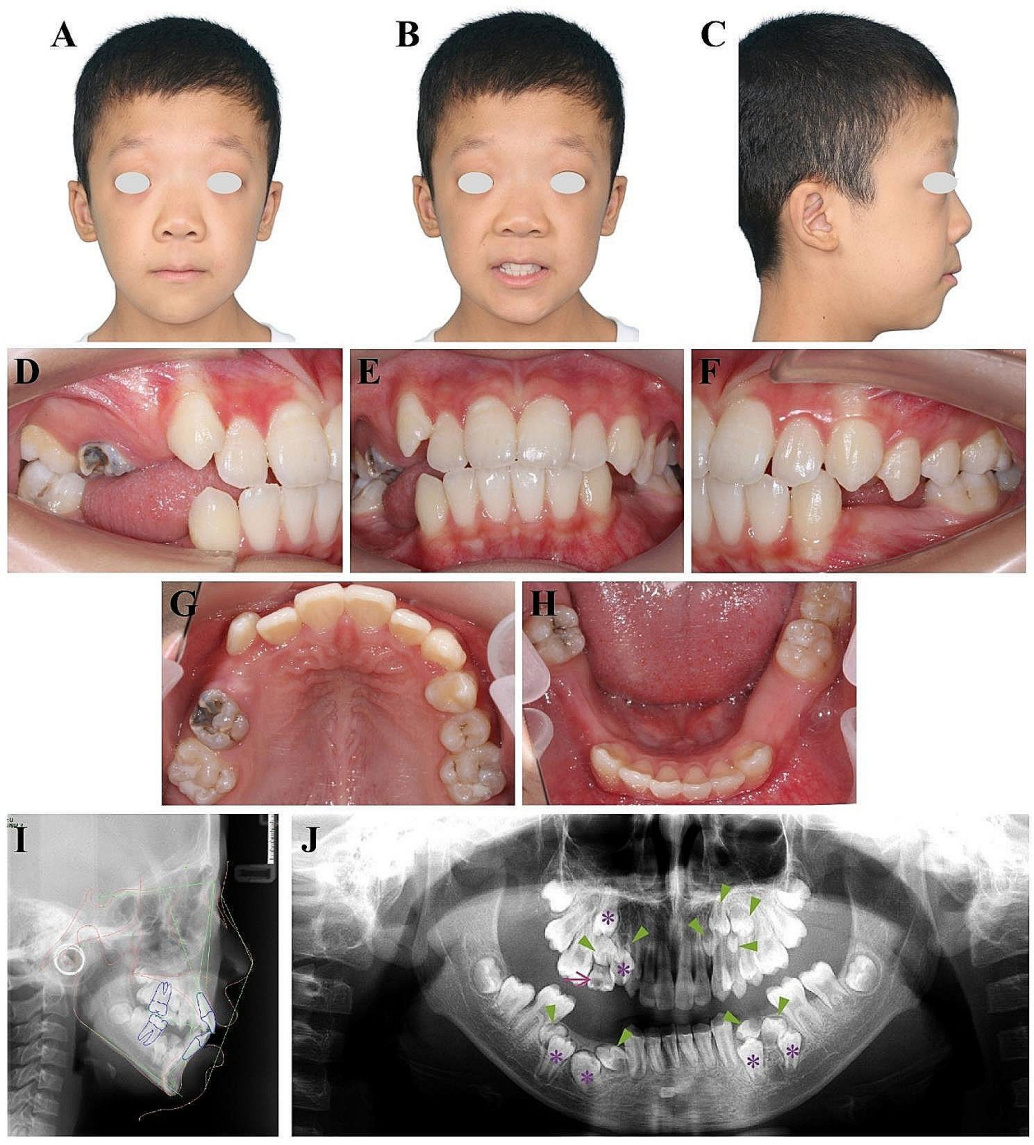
Pretreatment examinations of the patient with multiple supernumerary and impacted teeth. (A-C) Pretreatment facial photographs. (D-H) Pretreatment intraoral photographs. (I) Pretreatment lateral cephalogram. (J) Pretreatment panoramic radiograph. Impacted permanent teeth are denoted by purple asterisks. Supernumerary teeth are indicated by green arrowheads, while the retained deciduous tooth is indicated by the purple arrow
